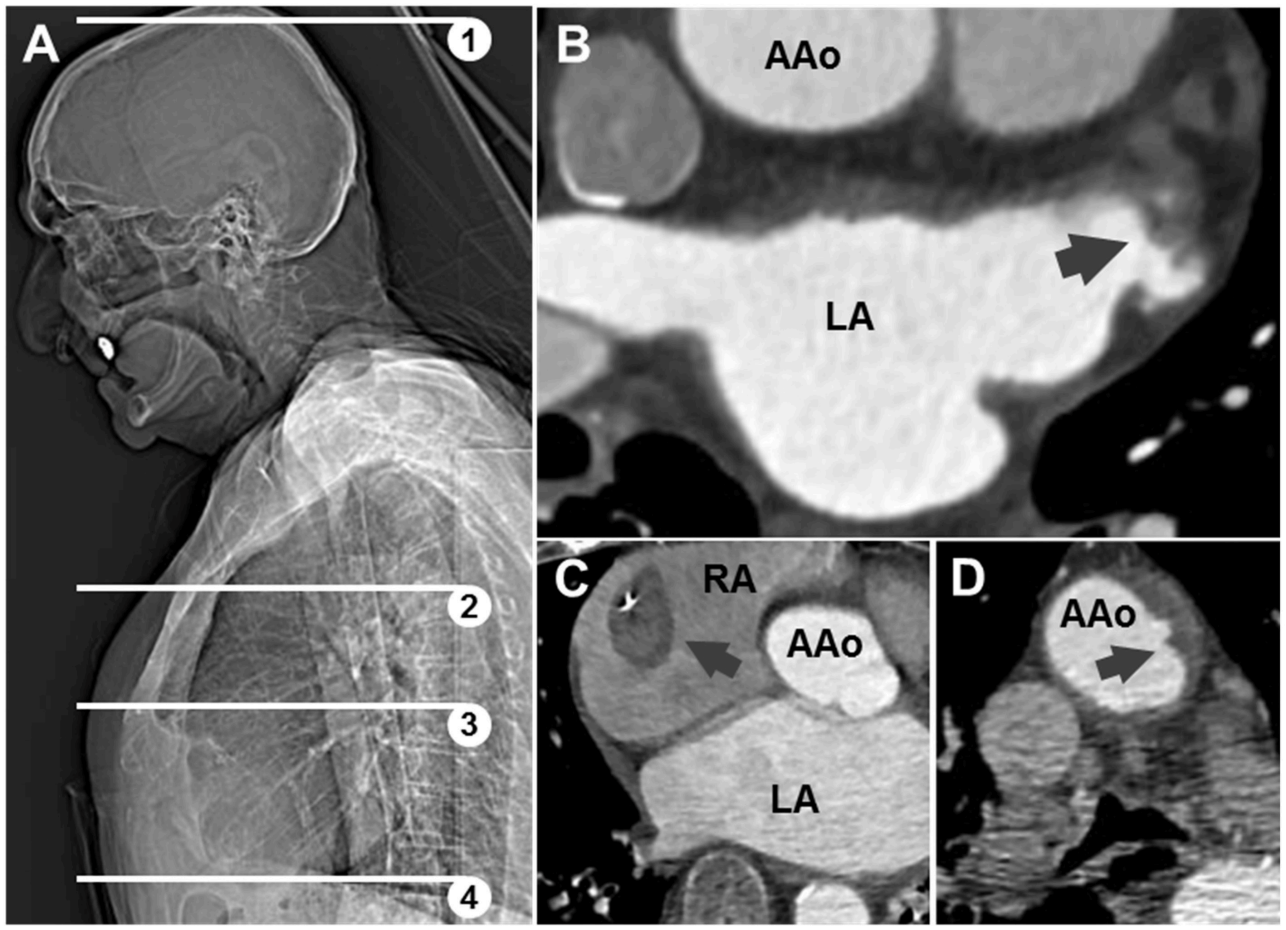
(A) Scout-view showing scan range of conventional (1,2) and extended (1–4) angiography; (3) corresponds to panel (B). Axial CT images: (B) thrombotic material in appendage of left atrium (LA) in patient 15; (C) thrombus around pacemaker lead in right atrium (RA) in patient 17; (D) ulcerating plaque in ascending aorta (AAo) in patient with ESUS.
